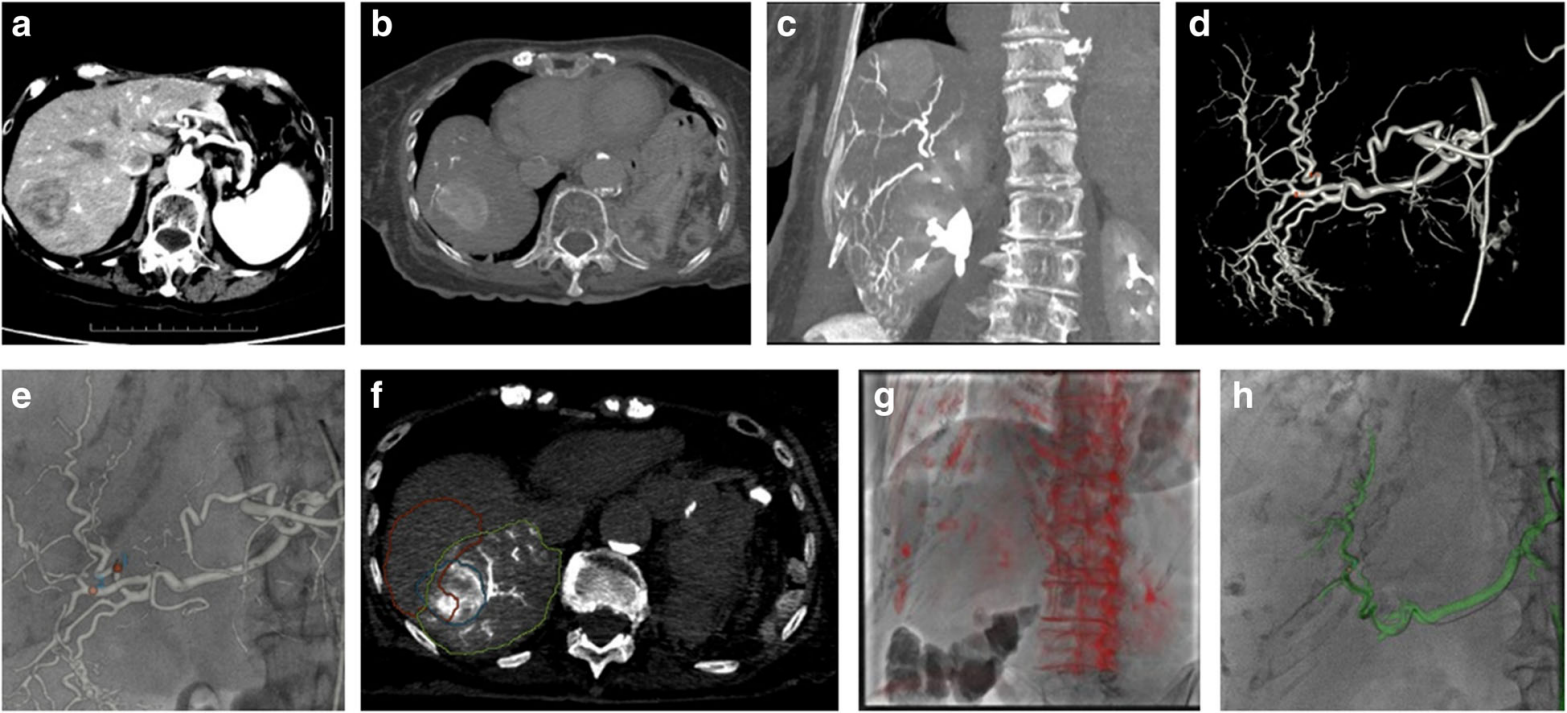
Example of CT usage during a Y90 procedure: The patient was a 90 year old female with a history of high-grade leiomyosarcoma of the gastric wall resected 3 years ago. A heterogeneous mass of 4.0 × 3.2 cm appeared in segment 7 on follow-up imaging (a) and the patient was referred to interventional radiology for SIRT Y90 treatment. A catheter- injected CT was performed from the proper hepatic artery, and anatomy was reviewed in reformatted views (b, c). A 3D model of the vessels was automatically generated and vessels were marked with potential MAA injection points (d, arrows), which were then overlaid on the fluoroscopy for guidance (e). An additional catheter-injected CT was performed from the desired location, which was then used to compute the tumor and perfused parenchyma volumes (f). The day of the Y90 treatment, registration of the same dataset using bony landmarks (g) was initially performed and the desired vessel was displayed for guidance (h)
